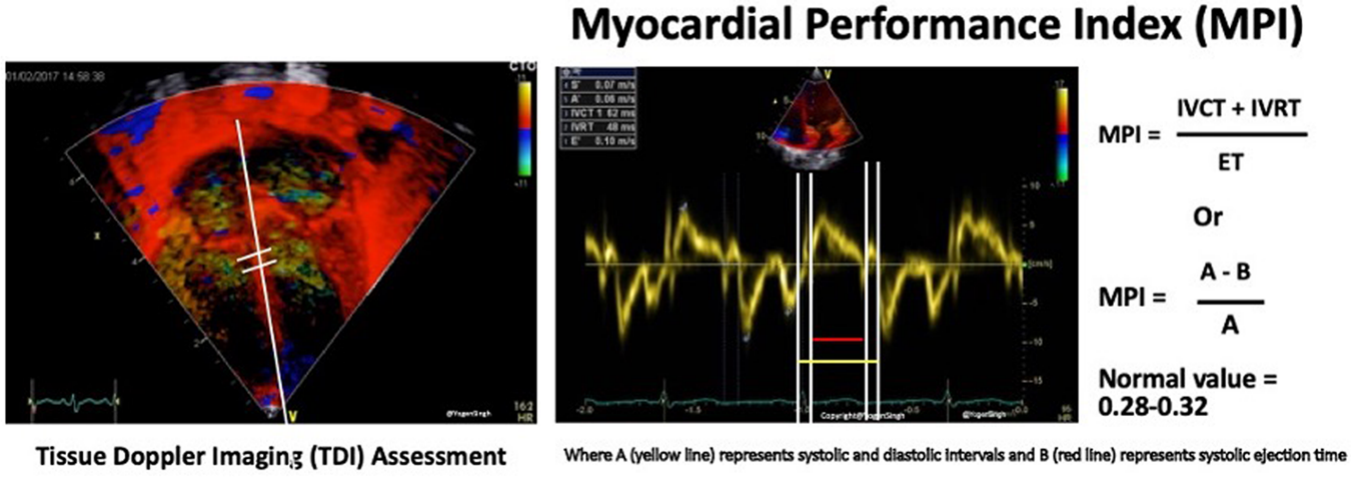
Tissue Doppler imaging (TDI, *left*) assessment of myocardial performance index (MPI, *right*).
